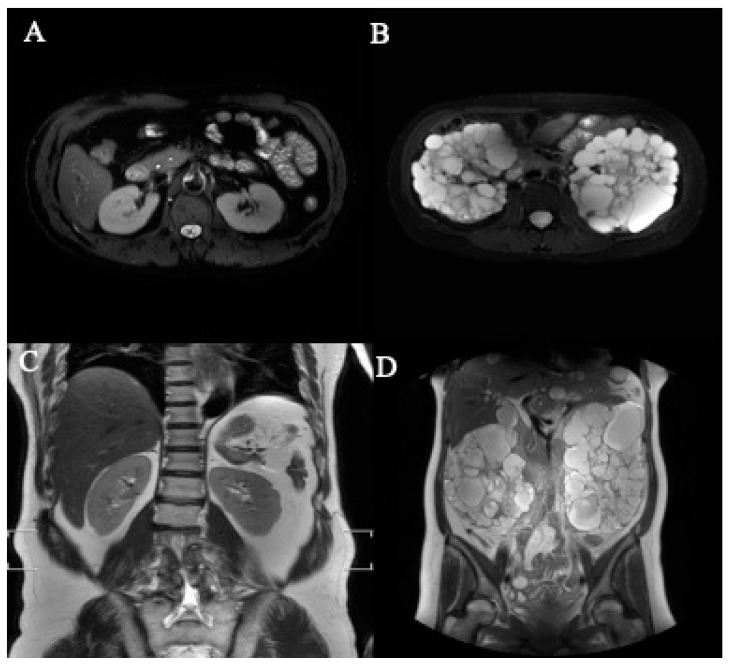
The representative axial- and coronal-section MRI images of kidneys. (A) Normal kidney (axial section), (B) polycystic kidney (axial section), (C) normal kidney (coronal section), (D) polycystic kidney (coronal section).
